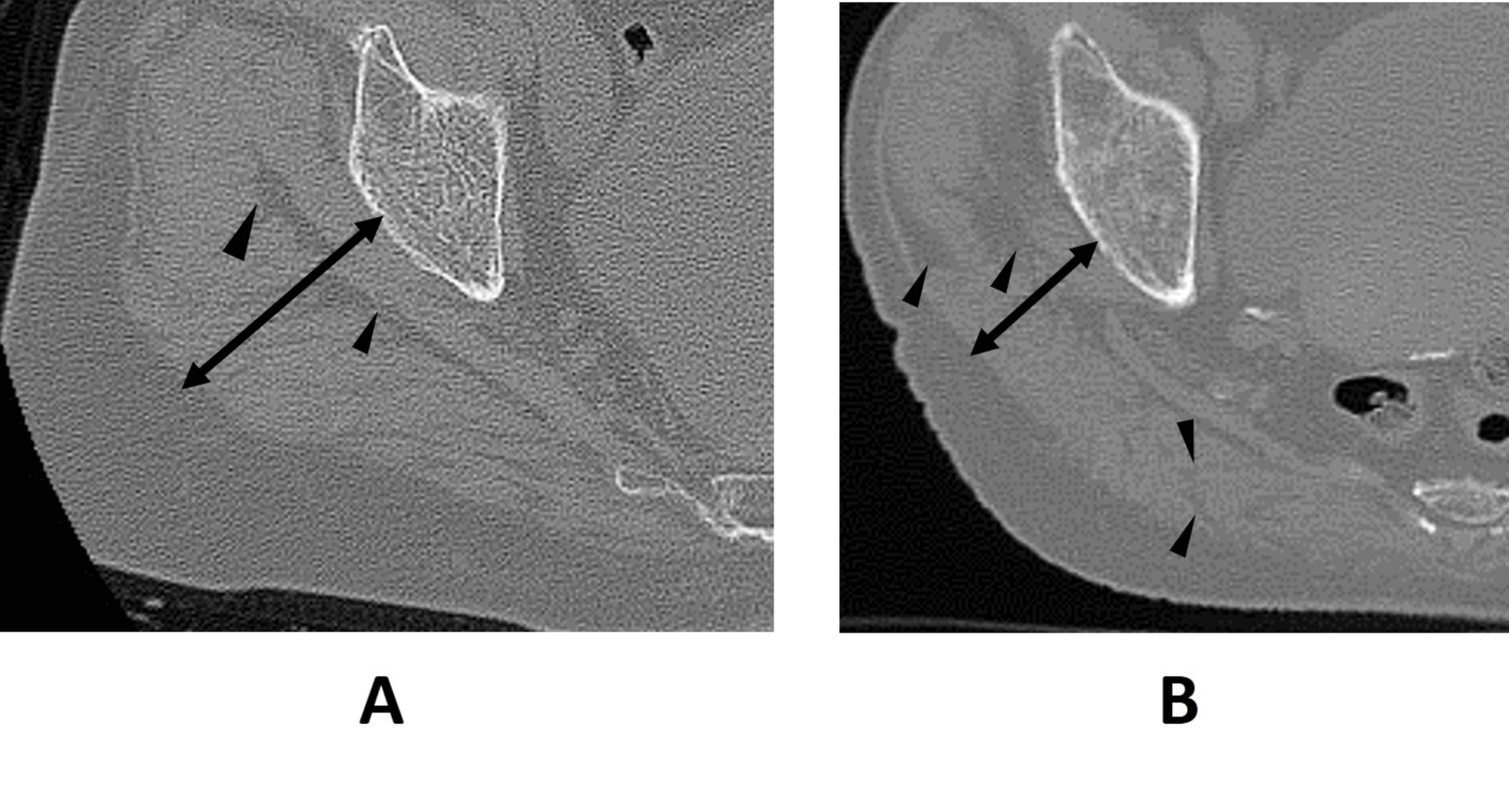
Case presentation Comparison of two axial CT images showing (A) robust muscle with minimal fatty infiltration in a stable fracture and (B) thinner muscle with substantial fat accumulation in an unstable fracture. The image suggests that sufficient muscle mass may help mitigate the impact on the proximal femur. Double-headed arrow: muscle thickness covering the bone; arrowheads: inter- and intra-muscular fat tissue.
